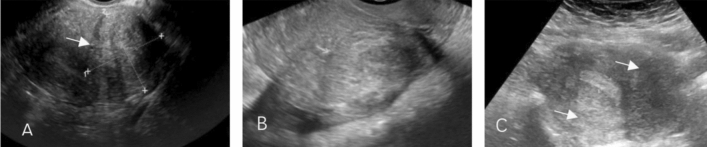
Two-dimensional transvaginal ultrasound images of adenomyosis. (A) with anterior adenomyosis; (B) with posterior adenomyosis; (C) with both anterior and posterior adenomyosis.
